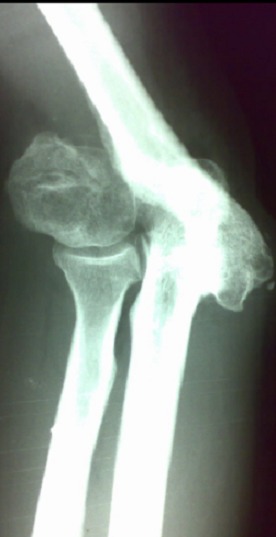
Radiographie de face montrant une fracture luxation du coude avec cal vicieux du condyle huméral externe
